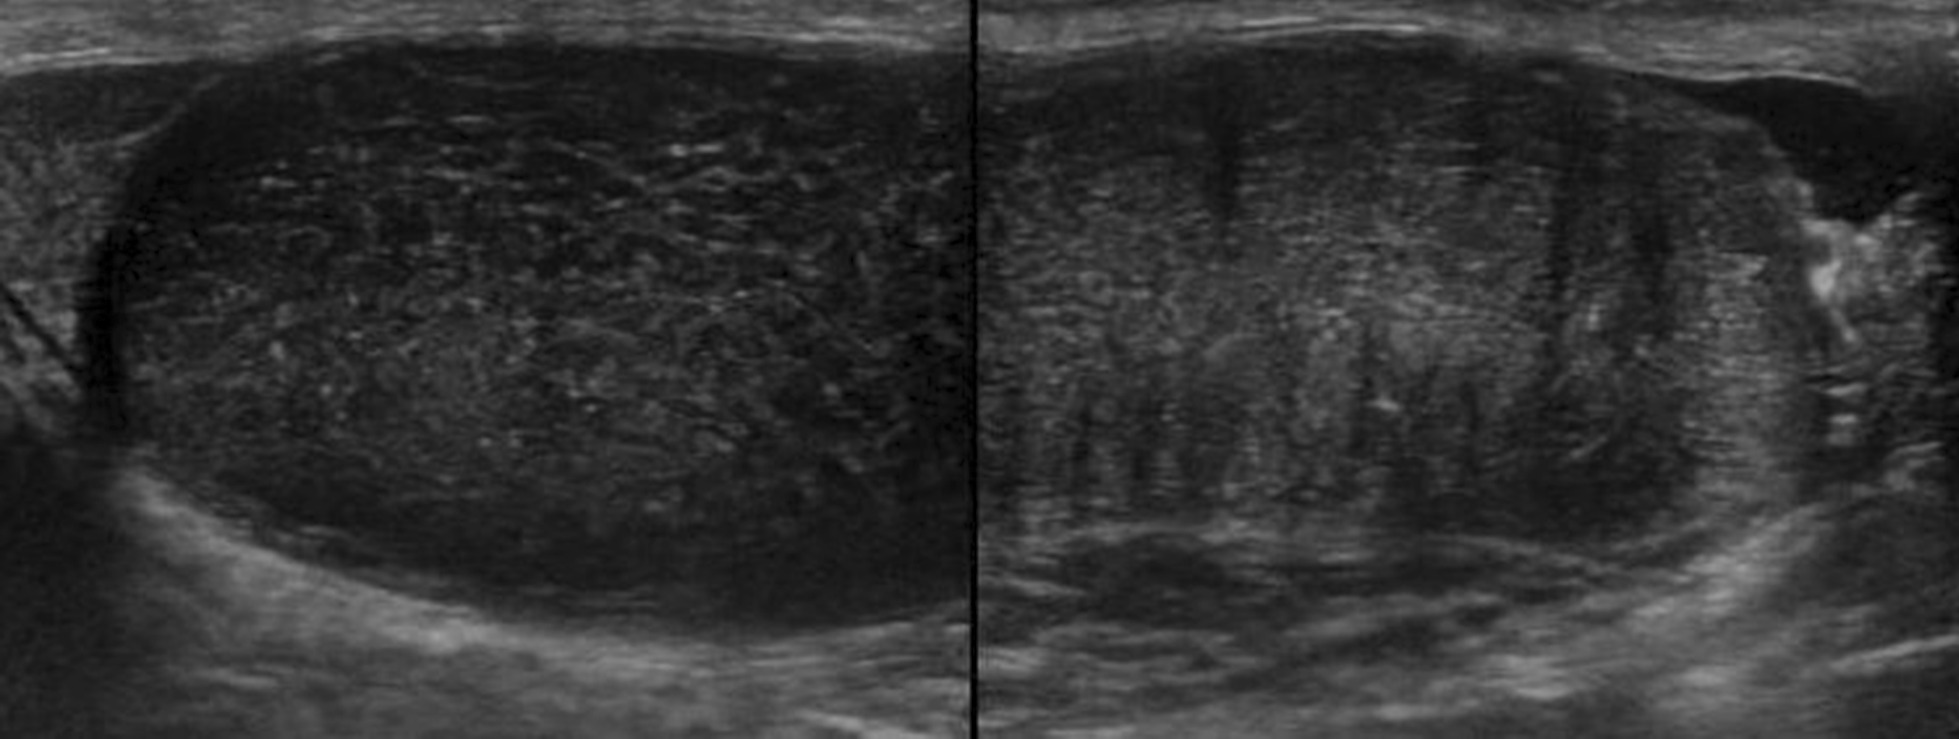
Ultrasonography of bilateral testes showing similar echogenicity
